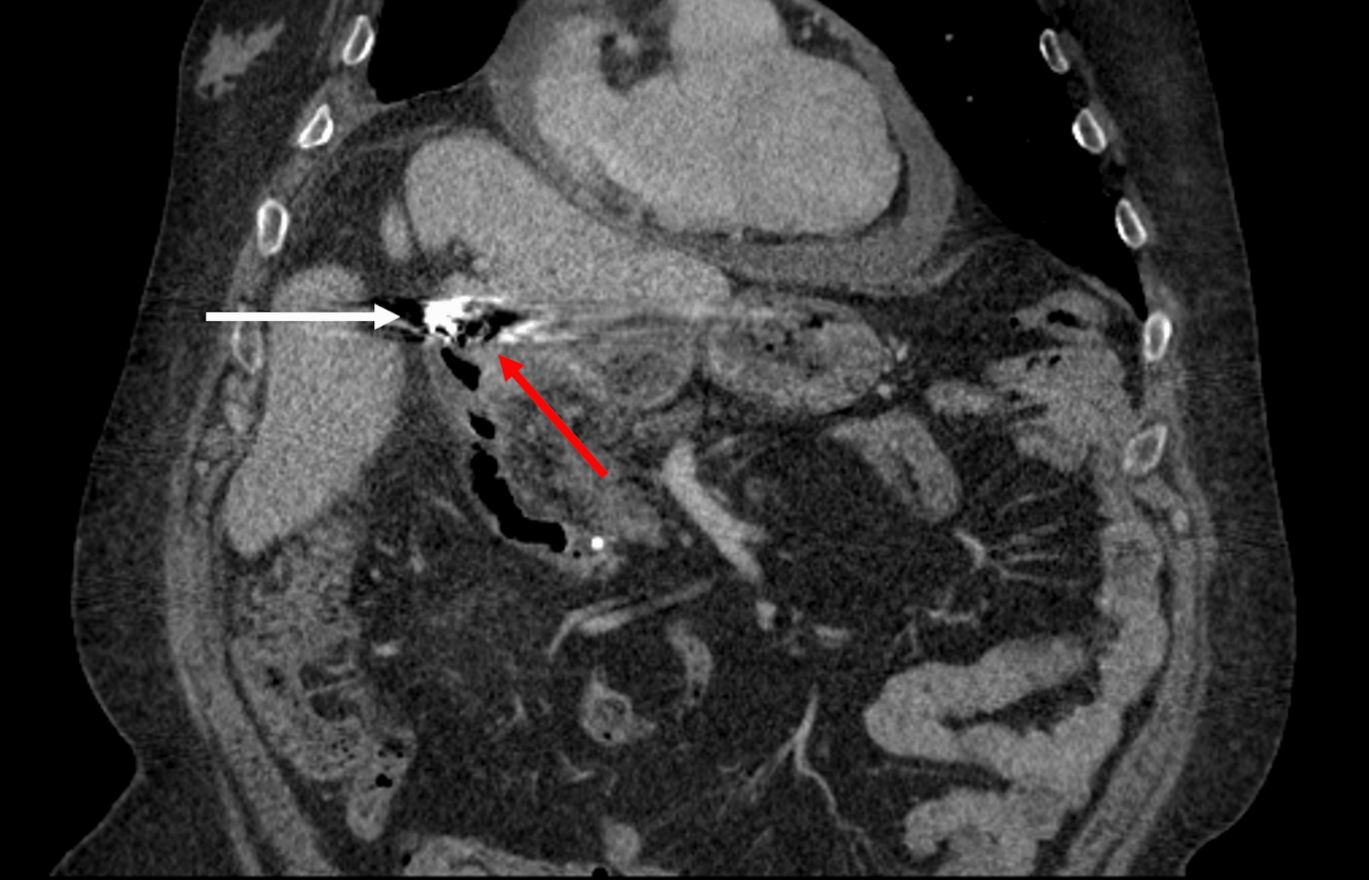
Coronal CT immediately after the transcatheter arterial embolization procedure showing the embolization coils (white arrow) in close proximity to the duodenal bulb (red arrow).
